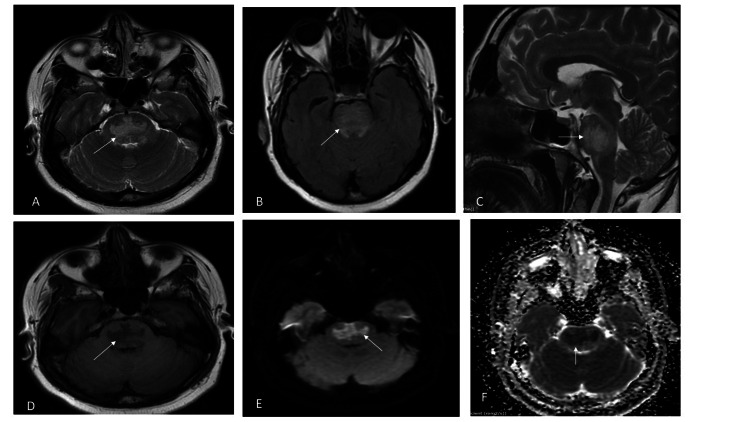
An MRI of the brain demonstrates a trident sign. The T2 axial (A), T2 FLAIR axial (B), and T2 FLAIR sagittal (C) images show a hyperintense signal in a trident shape involving the central pons (arrows). The T1 axial sequence (D) shows a hypointense signal in the pons (arrows). Diffusion-weighted and apparent diffusion coefficient sequences (E and F, respectively) show diffusion restriction in a trident shape involving the central pons (arrow). FLAIR: fluid-attenuated inversion recovery
